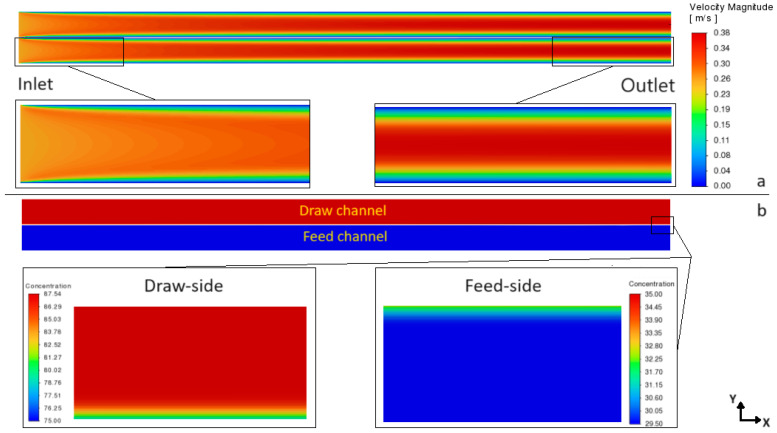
(a) Velocity contours and (b) concentration contours, at the middle longitudinal section of module in CTA–FO #2 case.
